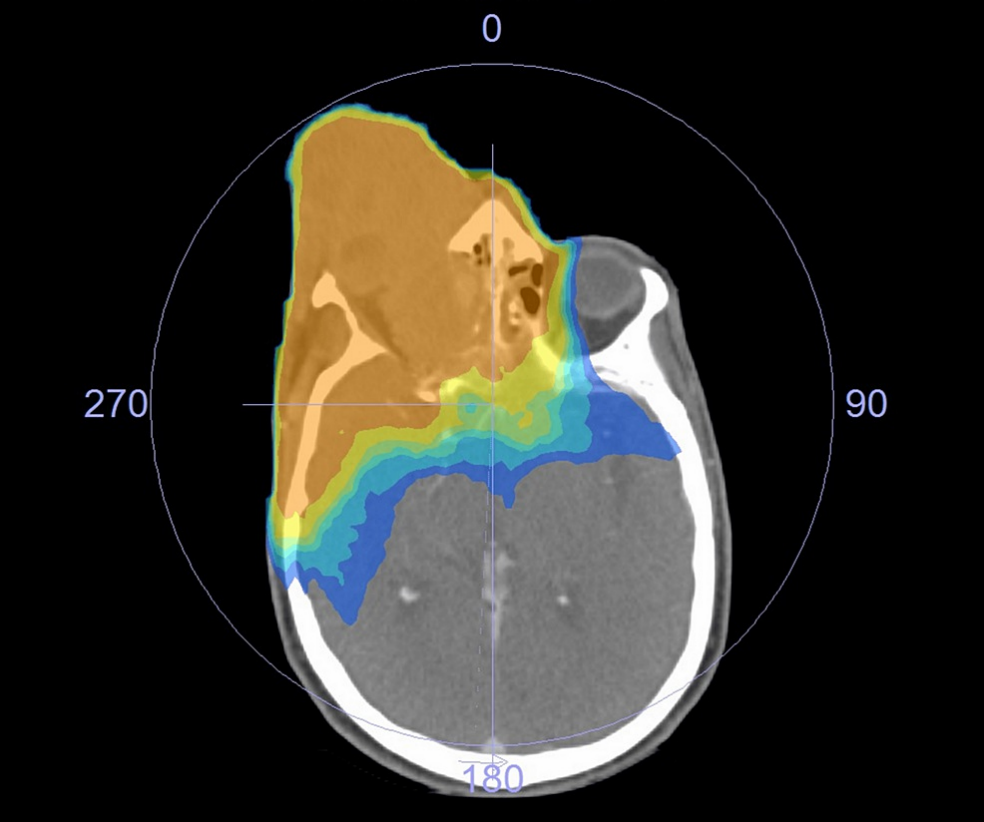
Radiotherapy isodose curve (axial view): the planning CT scan with 60 Gy (in yellow) and 70 Gy (in orange) isodose lines.
